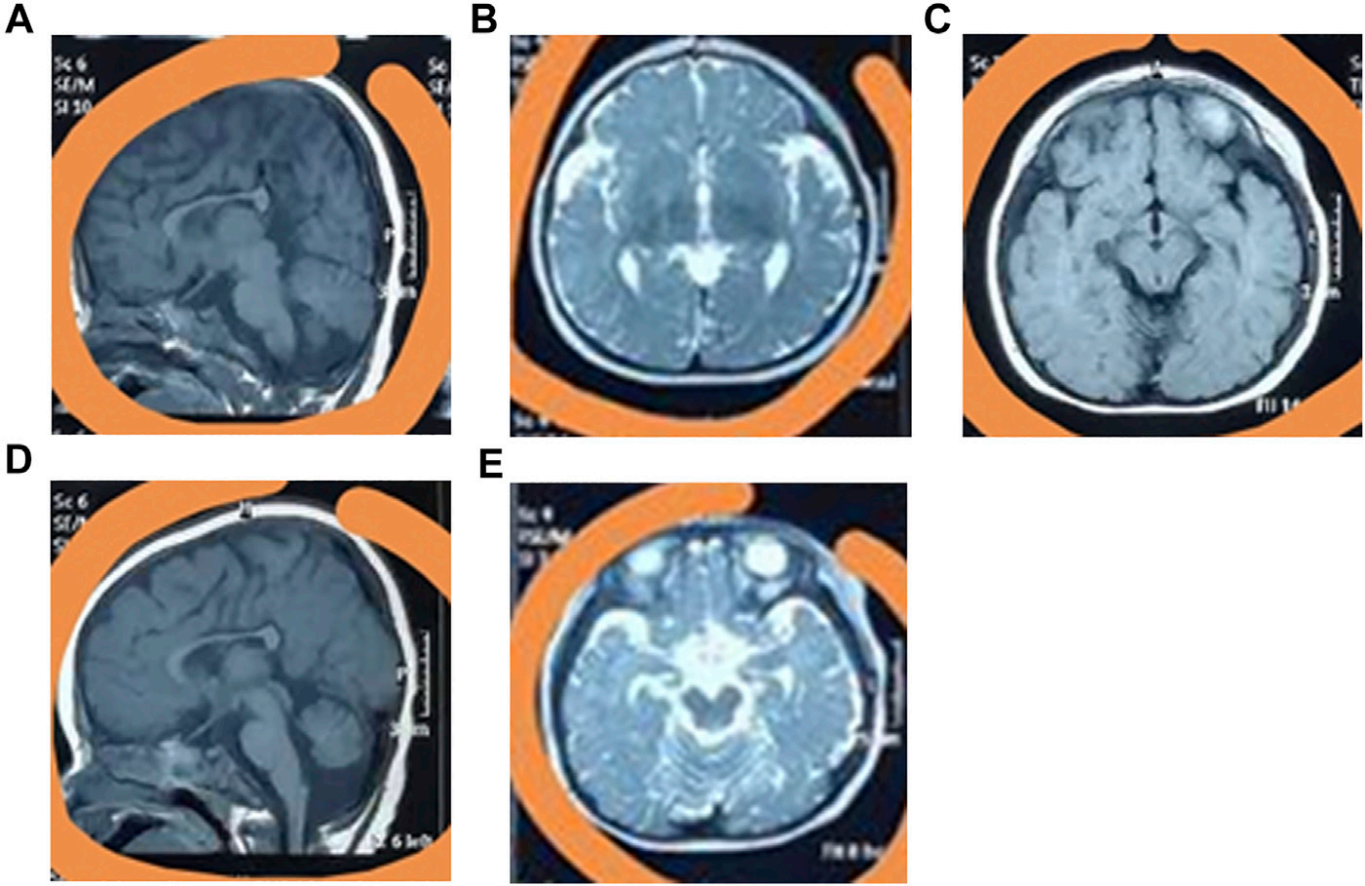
The clinical phenotypes of the patient. The MRI testing identified the overt carcass dysplasia (A), bilateral forehead subarachnoid space widening (B), right iliac choroidal fissure cyst (C), large cisterna magna (D), and cerebellar sulcus widening (E).
